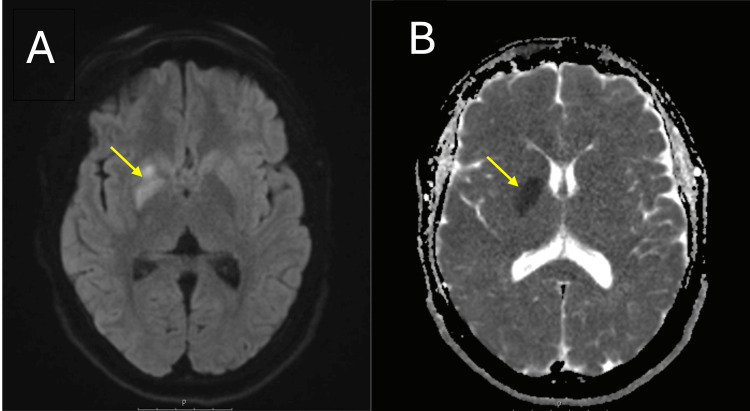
MRI of the head. (A) Diffusion-weighted imaging demonstrating restricted diffusion in the right lentiform nucleus, predominantly involving the globus pallidus, consistent with acute infarction. (B) Apparent diffusion coefficient map showing corresponding hypointensity, confirming true restricted diffusion.
